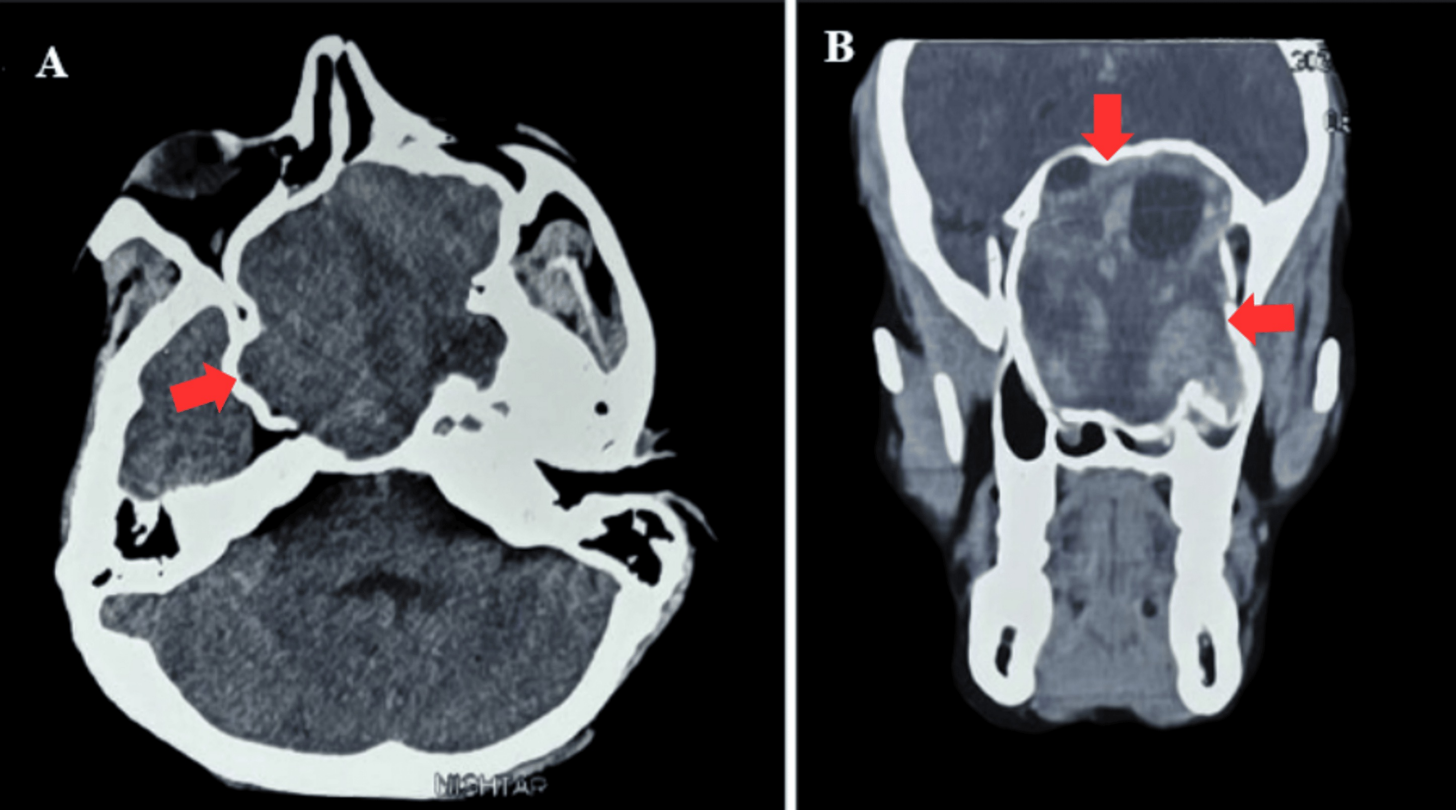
Axial CT image (A) showing skull base mass (red arrow) compressing the adjacent structures. Coronal CT image (B) showing proptosis caused by a mass involving bilateral nasal cavities and orbital floors (red arrows).
